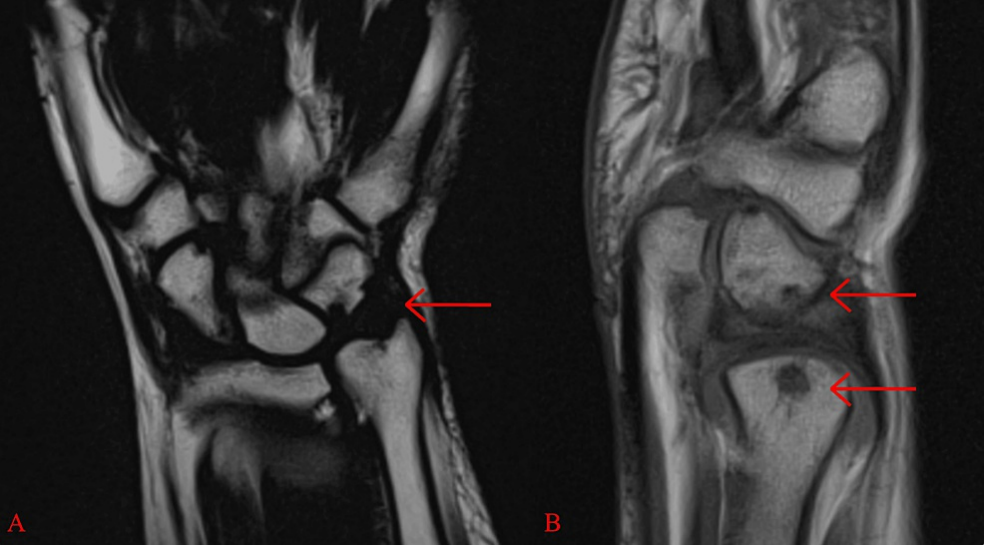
(A) T1-weighted coronal imaging reveals a positive ulnar variance of 2.9 mm and degenerative subcortical cyst formation of the proximal cortex of the triquetrum and the distal ulna. (B) T1-weighted sagittal imaging at the level of the triquetrum reveals moderately advanced degenerative signal changes of the distal ulna and the triquetrum due to chronic ulnotriquetral impaction. TFCC = triangular fibrocartilage complex
